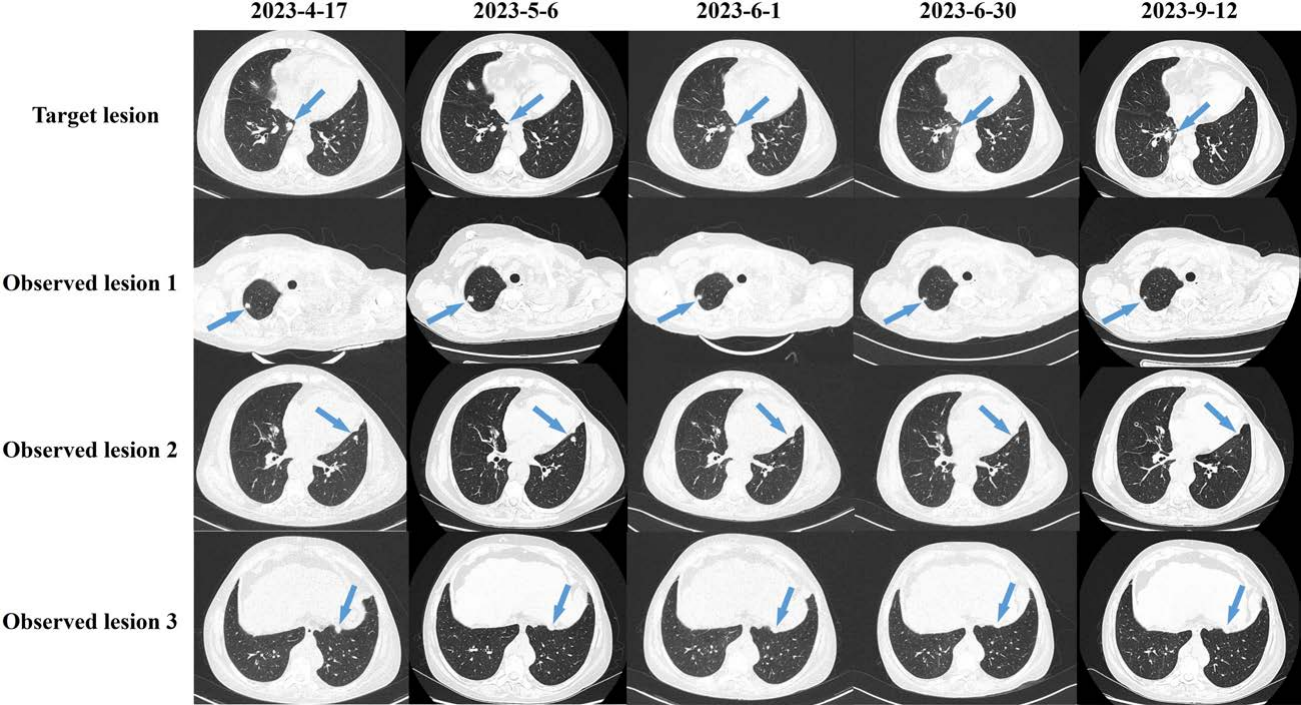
CT scans throughout the treatment. The target lesion is the site that was irradiated, and the observed lesions are the metastases without irradiation. The blue arrows indicate the lesion location.
